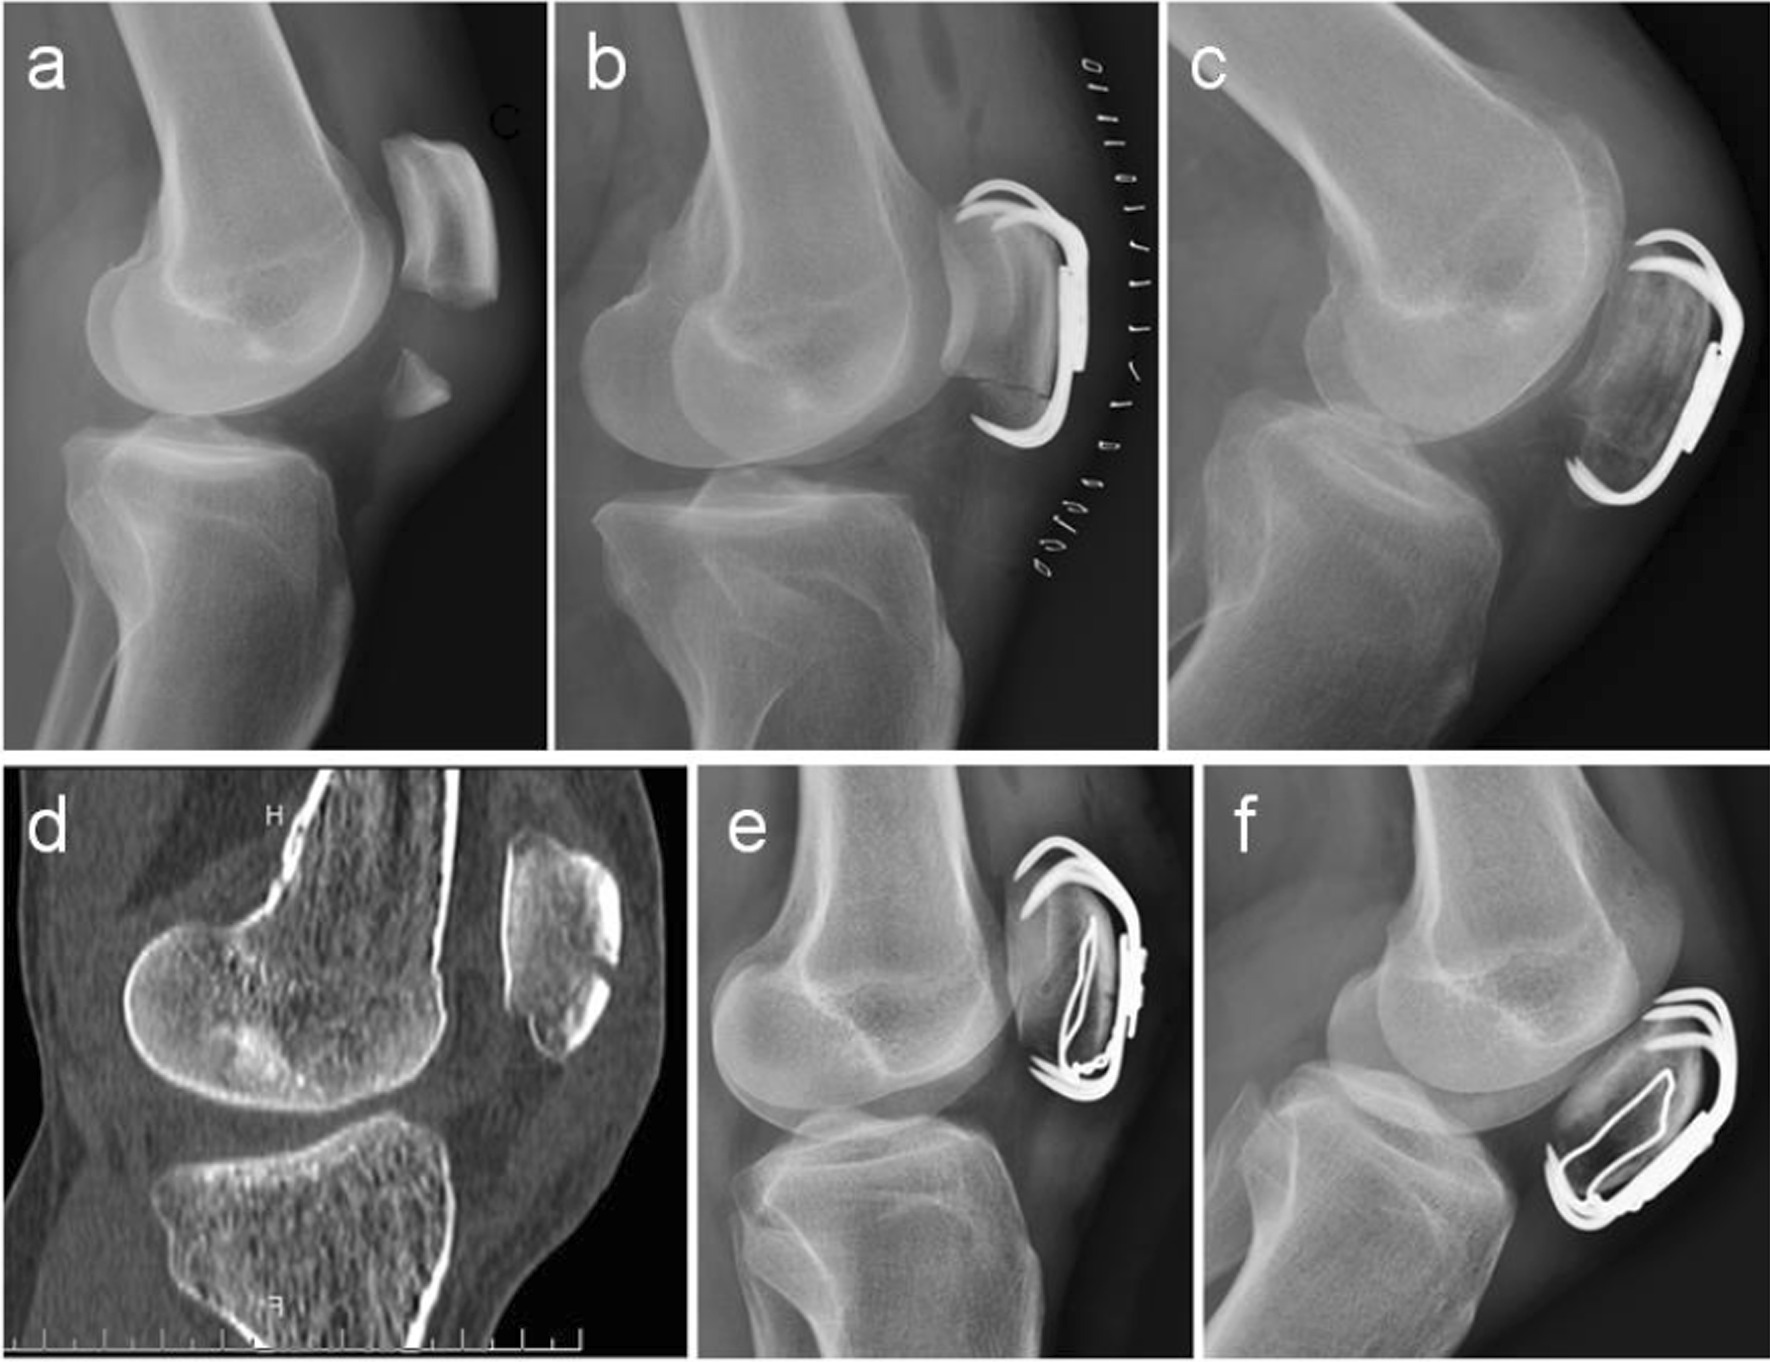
Comparison of postoperative fracture healing between the control and experimental patient cohorts a-d. A 42 year old male was admitted to the hospital for correction of fracture caused by a fall 4 h prior to admission. a. Pre-operative X-ray lateral film b. Post-operative X-ray lateral film c. Post-operative X-ray lateral film at the 12-week follow up d-f. A 38 year old female was admitted to the hospital for correction of fracture caused by a fall 3 h prior to admission. d. Pre-operative CT examination of the sagittal plane e. Post-operative X-ray lateral film f. Post-operative X-ray lateral film at the 12-week follow up
